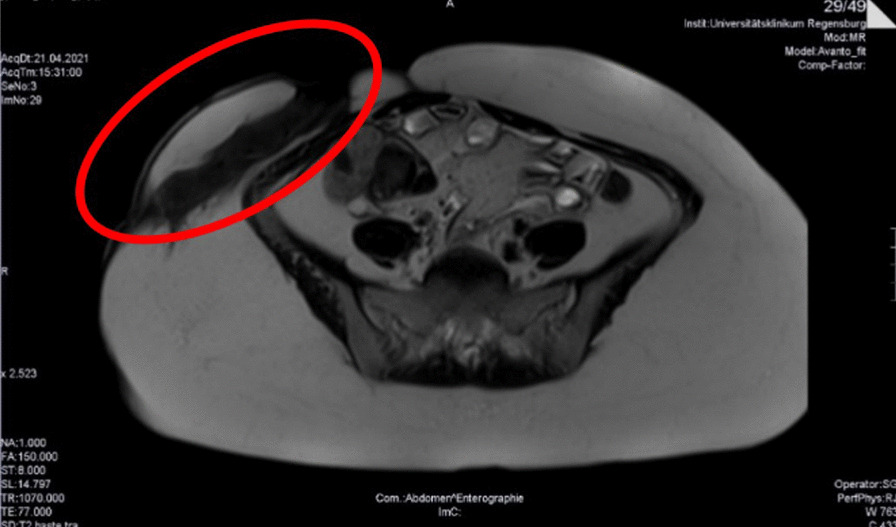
MRI enterography of the large subcutaneous abdominal fistula (red circle), April 2021
